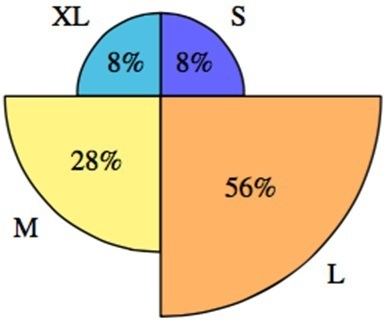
Retrospective audit showing the categories that patients would have been imaged in. 92% of patients would have had some form of dose reduction to their CBCT imaging regime.
